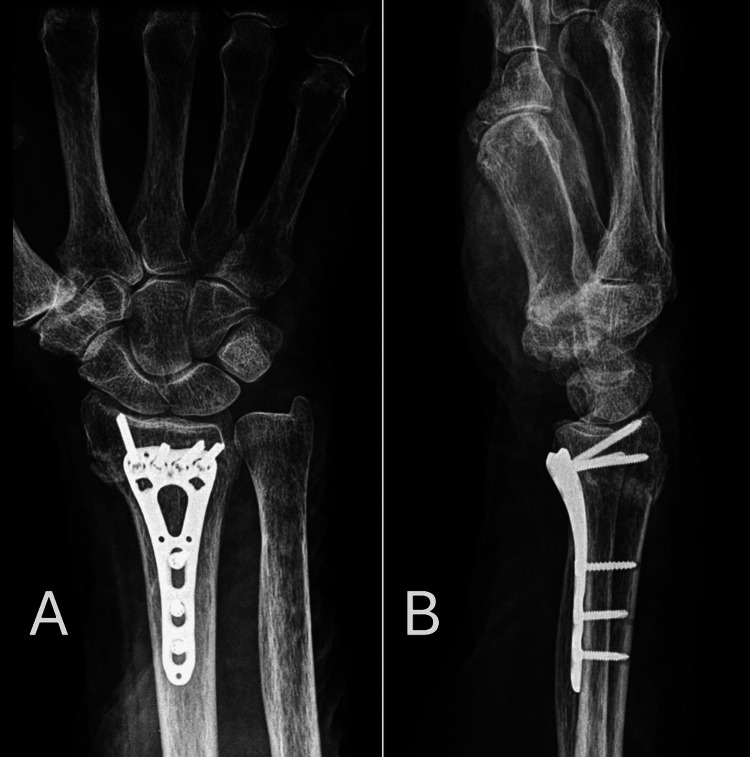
Anteroposterior (AP) and lateral views of the wrist X-ray with plate fixation. A: Anteroposterior (AP) view of the wrist X-ray with plate fixation. B: Lateral view of the wrist X-ray with K-wire fixation.
